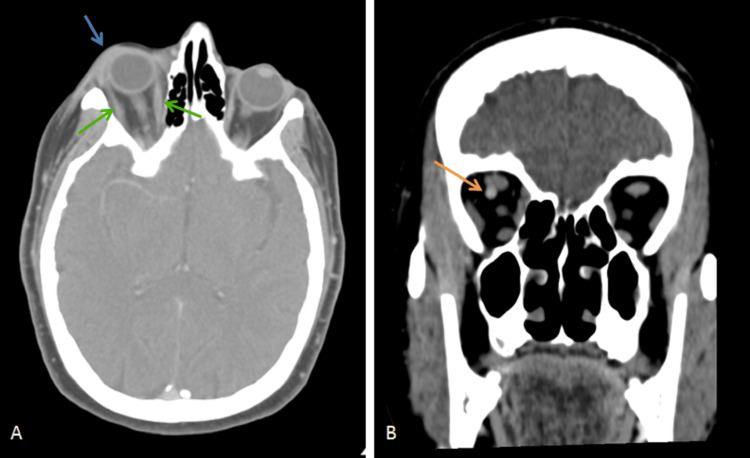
Orbital computed tomography images of the patient. (A) Axial view showing the inflammation of the upper right eyelid soft tissues and right eyeball proptosis (blue arrow), and the swelling of the medial and lateral rectus muscles (green arrows) and (B) coronal view showing the enlargement of the right superior ophthalmic vein (orange arrow).
